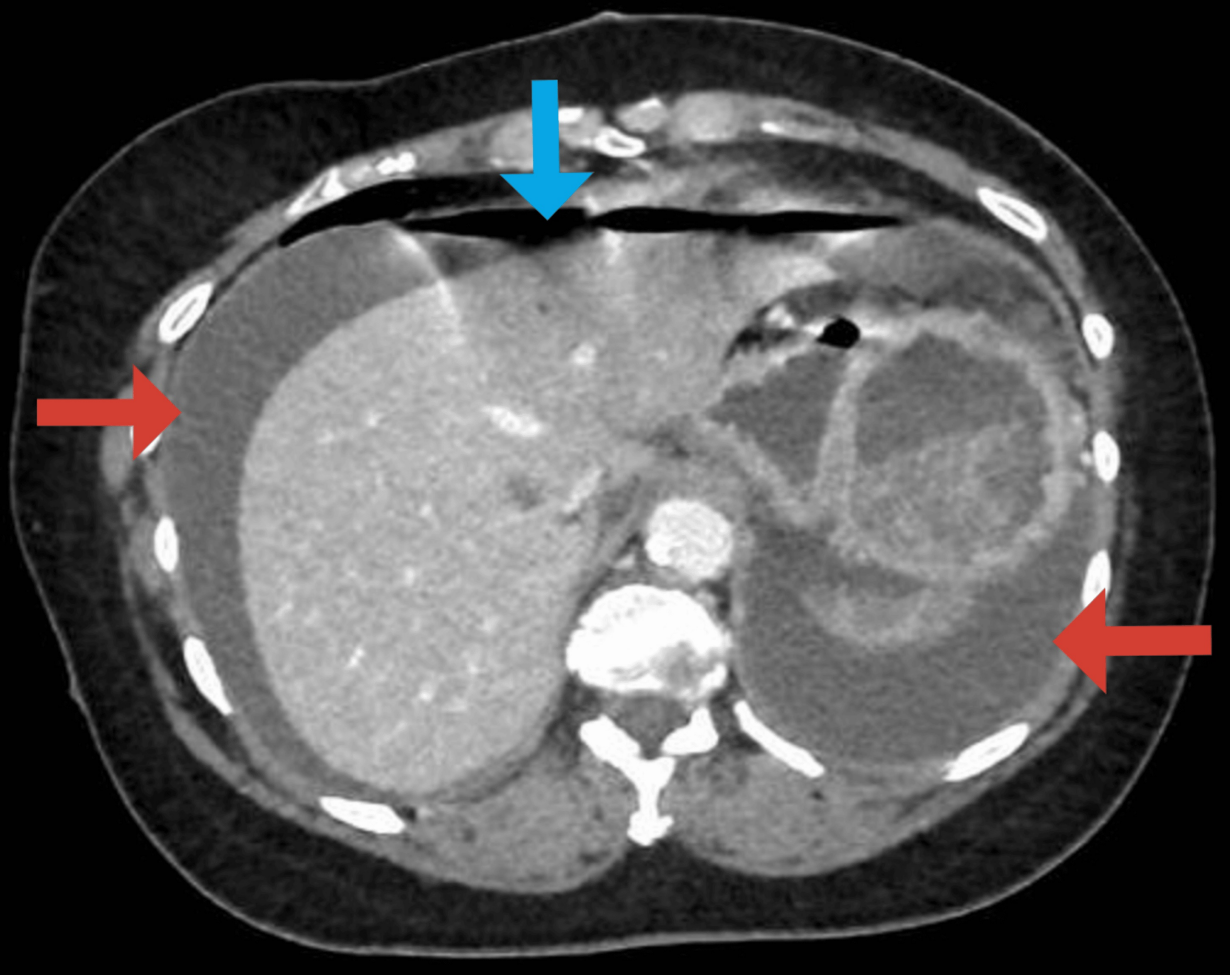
Contrast-enhanced abdominal computed tomography revealing pneumoperitoneum (bleu arrow) and significant peritoneal effusion (red arrows).
